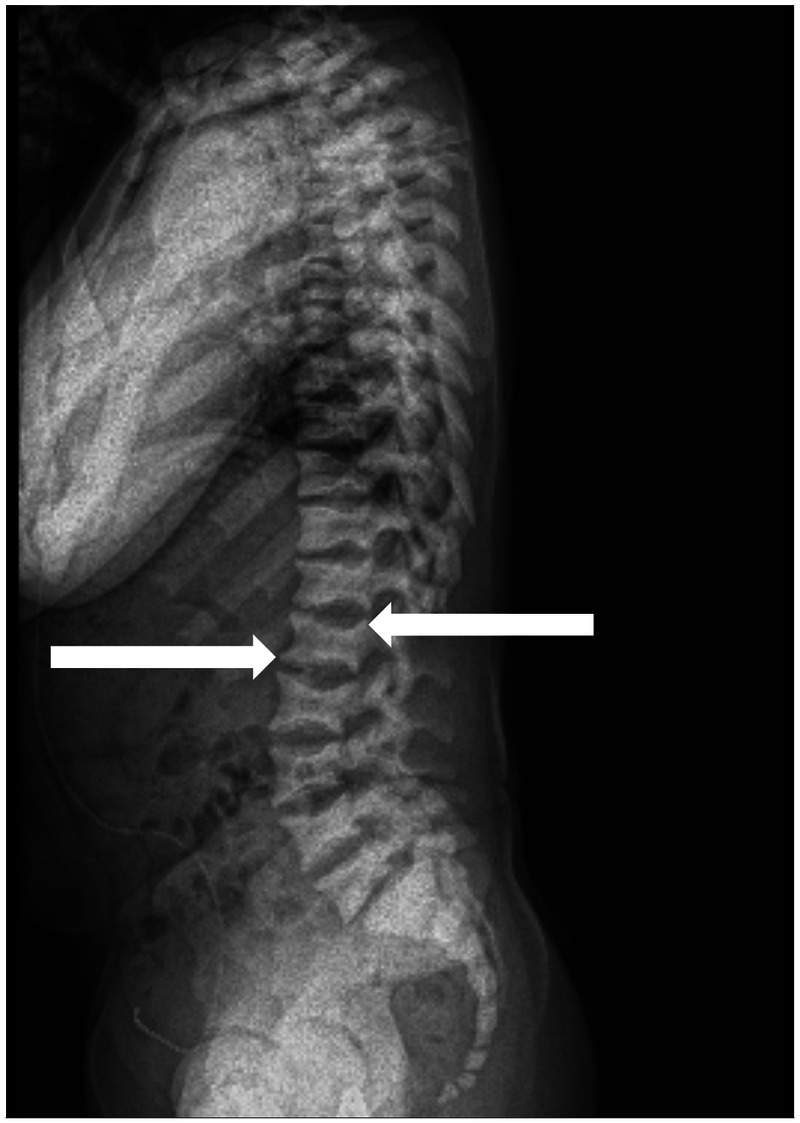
Lateral radiograph of a 23-year-old patient with OP (ADO II), showing the hyperdense sclerotic superior and inferior endplates of the vertebral bodies (white arrows), also termed “sandwich vertebrae”.
